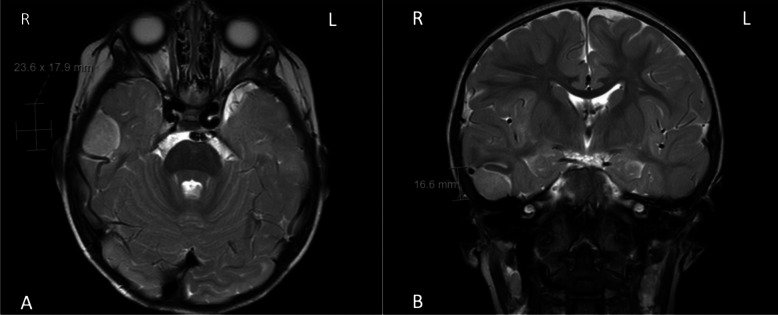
Axial (A) and coronal (B) T2-weighed MRI images demonstrate a rounded intradural, extra-axial lesion in the inferolateral temporal lobe with associated local mass effect and close association with the vein of Labbe. On contrasted images (not shown), the lesion homogeneously enhances
